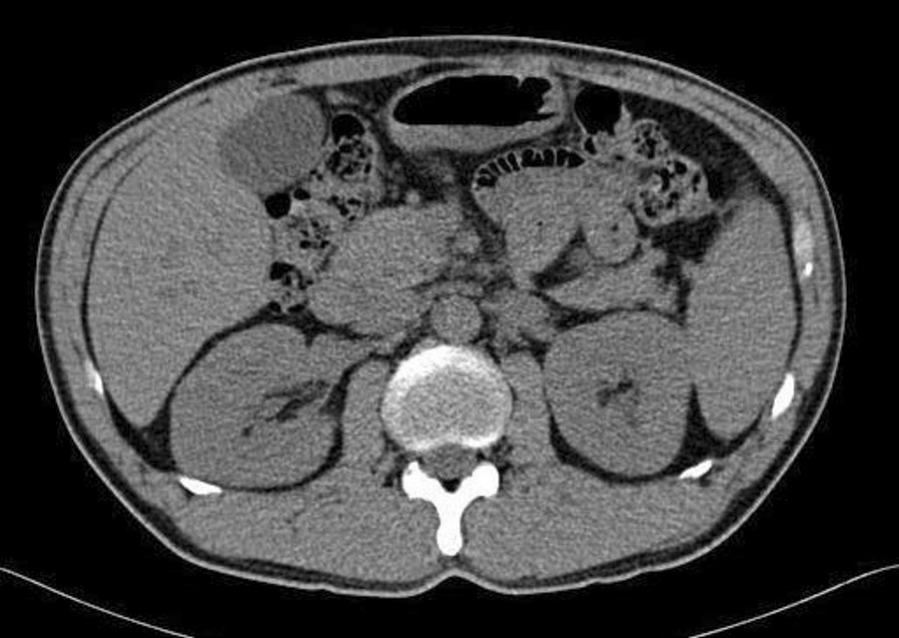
Non-contrast enhanced abdominal computed tomography showed both enlarged kidney and no hydronephrosis
